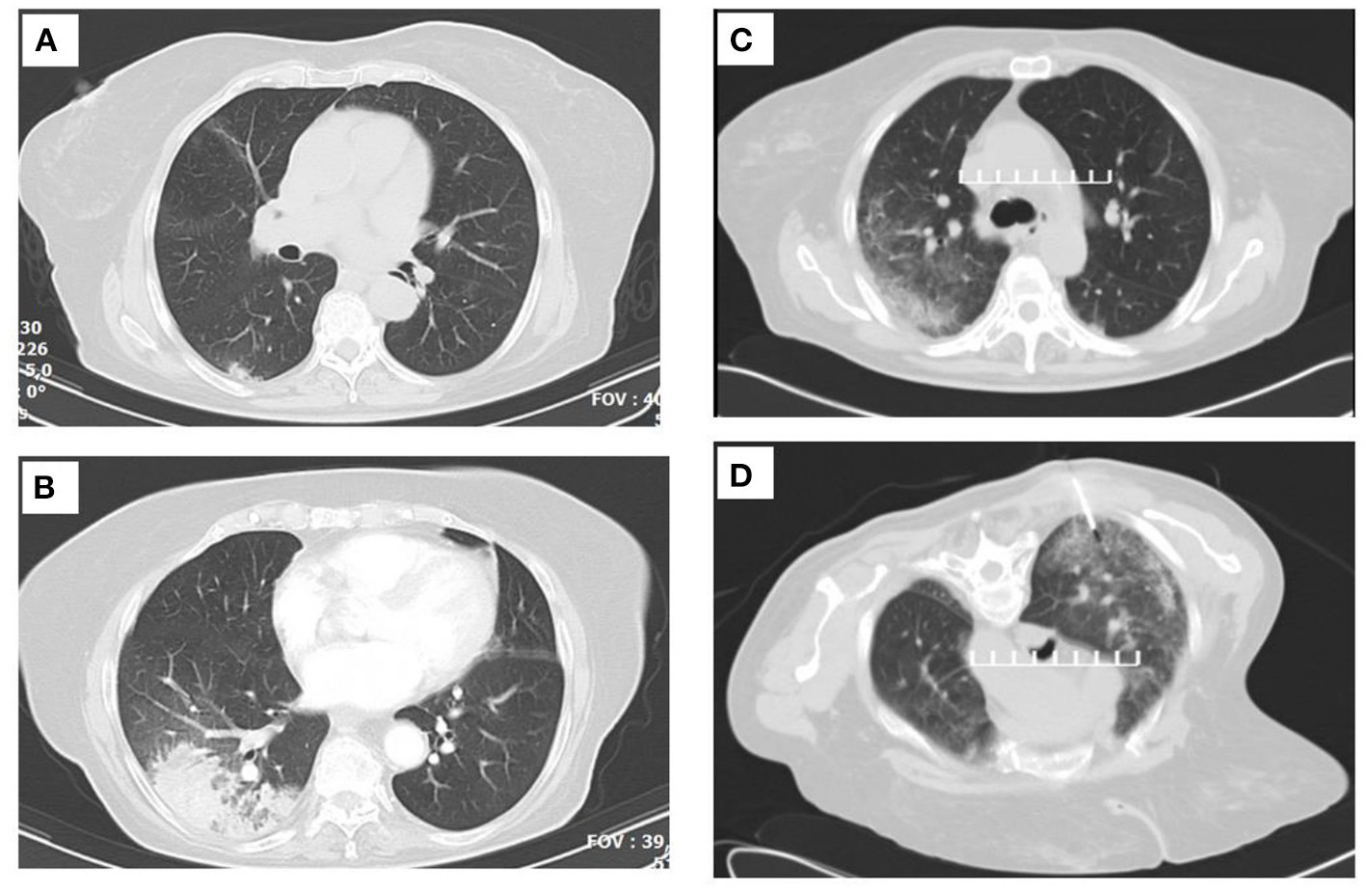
Serial thoracic computed tomographies (CTs) that demonstrate the lesion migration. (A) Image before corticosteroid initiation. (B) Image after the initial short-term corticosteroid treatment. (C) Image at the time of the CT-guided biopsy. (D) Fine-needle biopsy (FNB) cutoff point.
